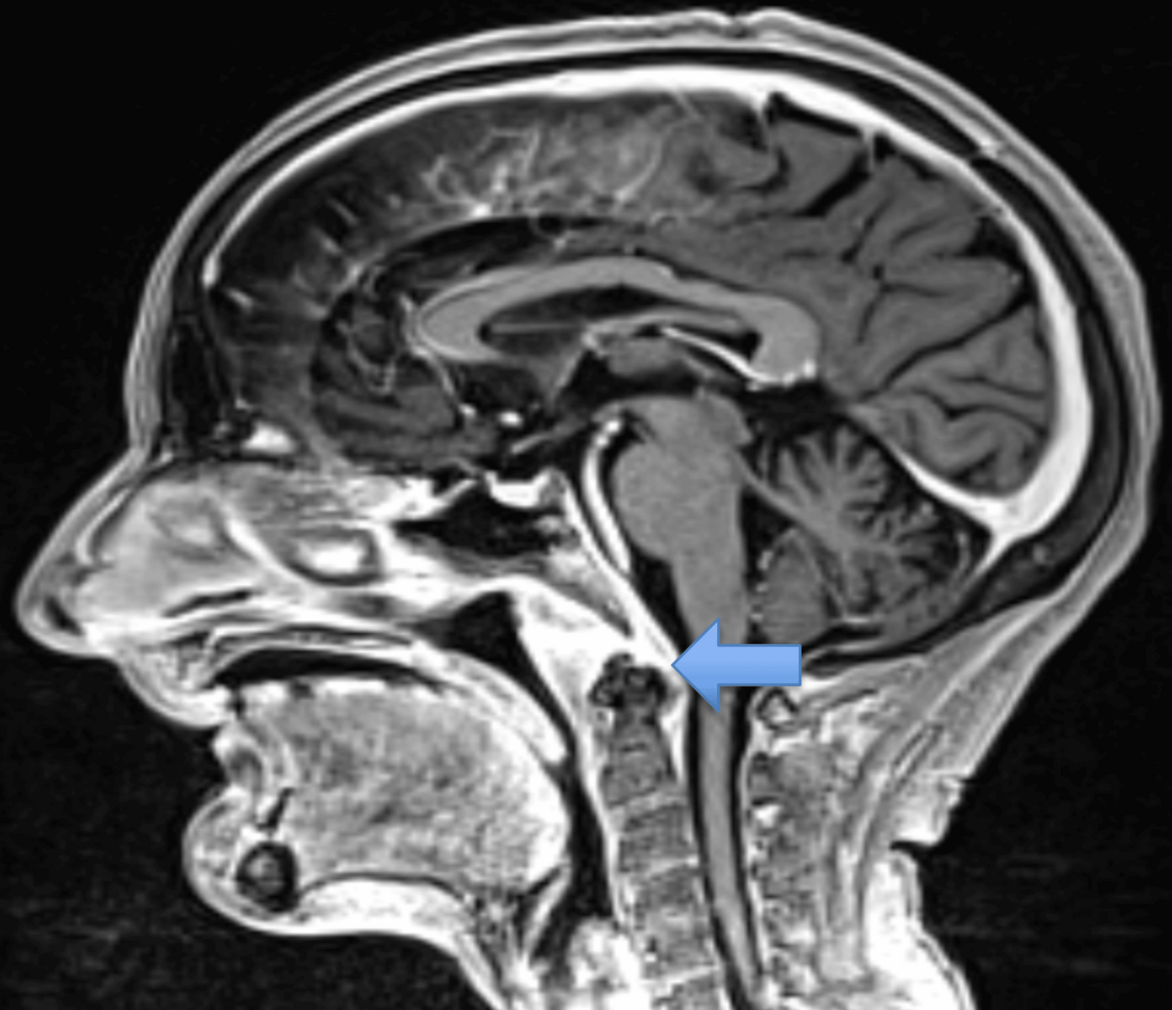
Contrast-enhanced MRI of the brain (T1-weighted, sagittal section) showing post-contrast enhancement of the C1 vertebra with degenerative changes and surrounding soft tissue enhancement. The blue arrow points to the degenerative changes in the C1 vertebra with surrounding soft tissue swelling and collection.
